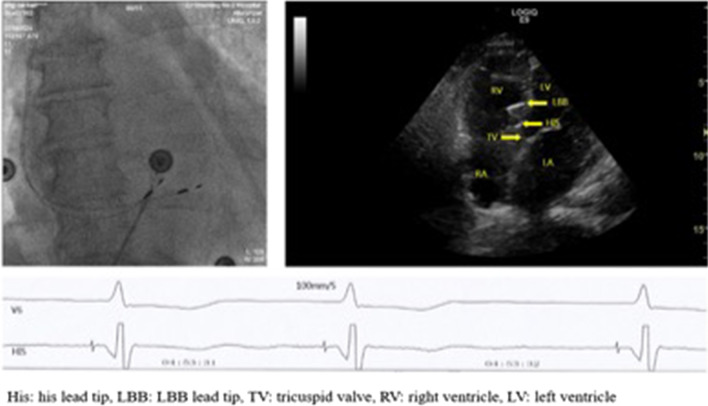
DSA image, 2D-Echocardiography mapping and electrophysiological intracavity map for patient case 29
